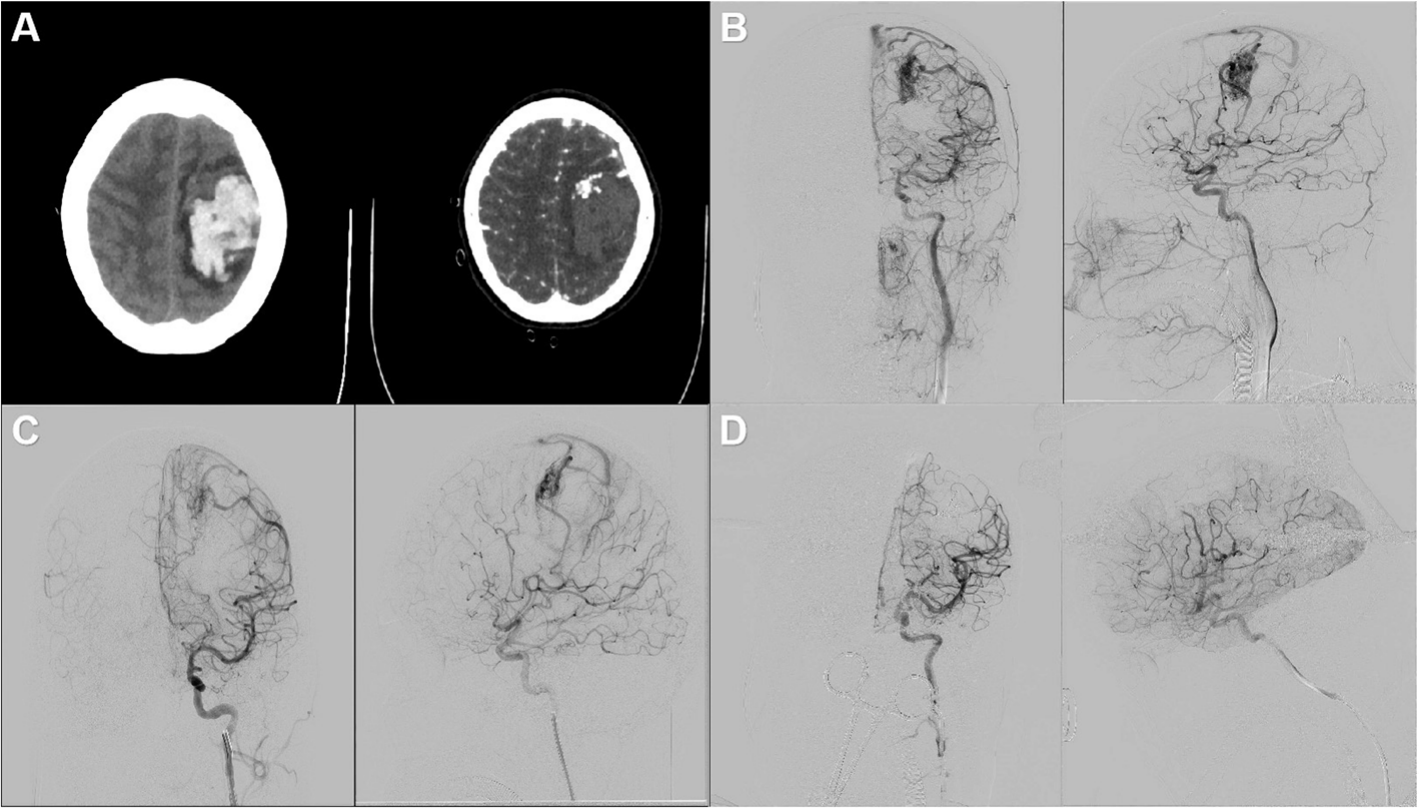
A Emergency CT scan indicates hemorrhagic lesions in the left frontal and parietal regions. Suspected left frontal vascular malformation with hemorrhage based on CTA original images. B DSA reveals a left frontal vascular malformation supplied by dual branches of the left middle cerebral artery and a single branch of the left anterior cerebral artery, with superficial venous drainage. C Embolization of branches supplying the AVM from the middle cerebral artery is performed. D Surgical resection and evacuation of the intracranial hematoma are performed. Intraoperative DSA confirms complete obliteration of the lesion post-resection
